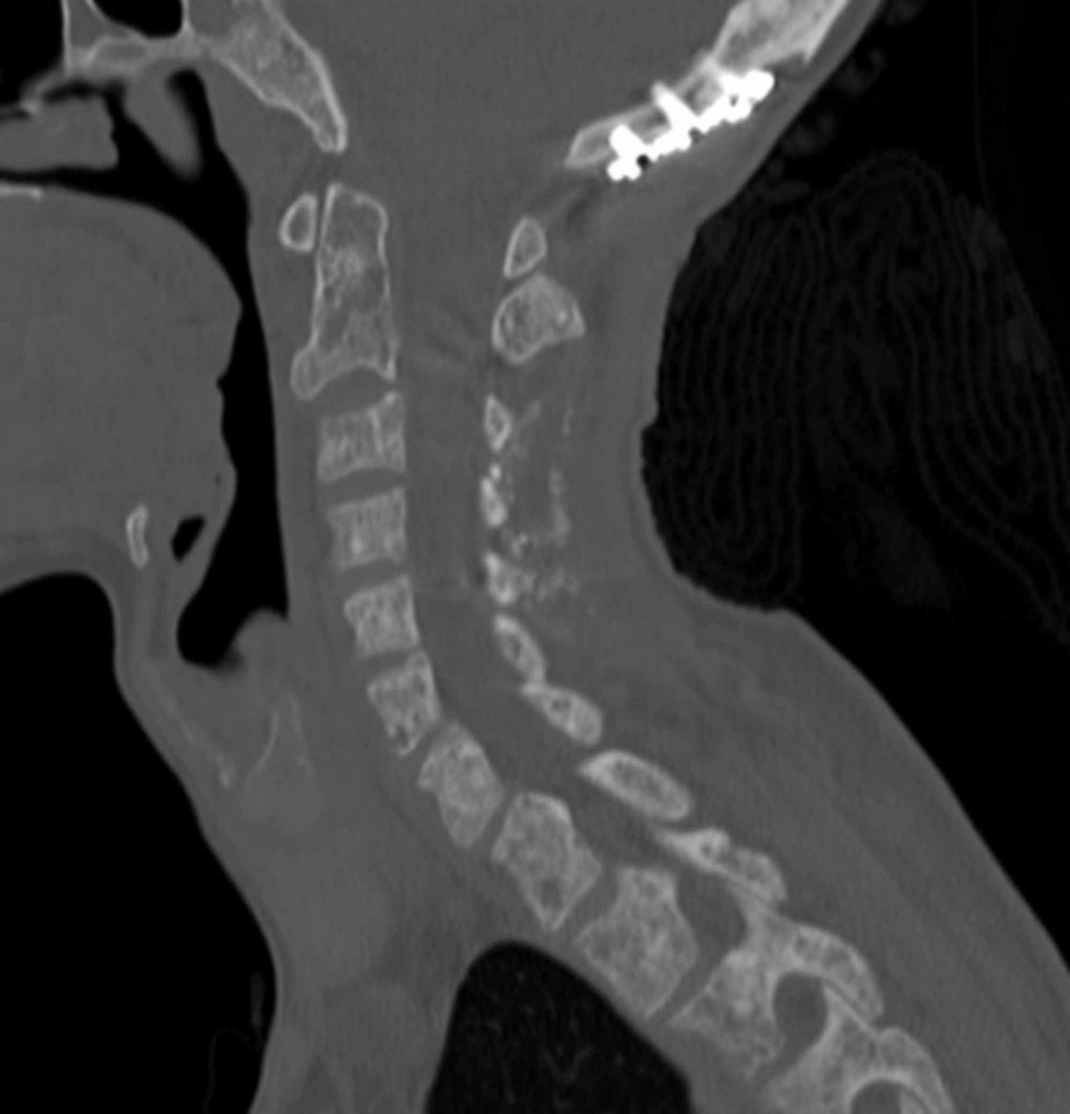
Postoperative CT of the cervical spine at one-year follow-up Follow-up CT of the cervical spine at one year showing posterior spinal fusion hardware from occiput to C5 showing appropriate alignment and reconstitution of bony integrity in the upper and subaxial cervical spine.
